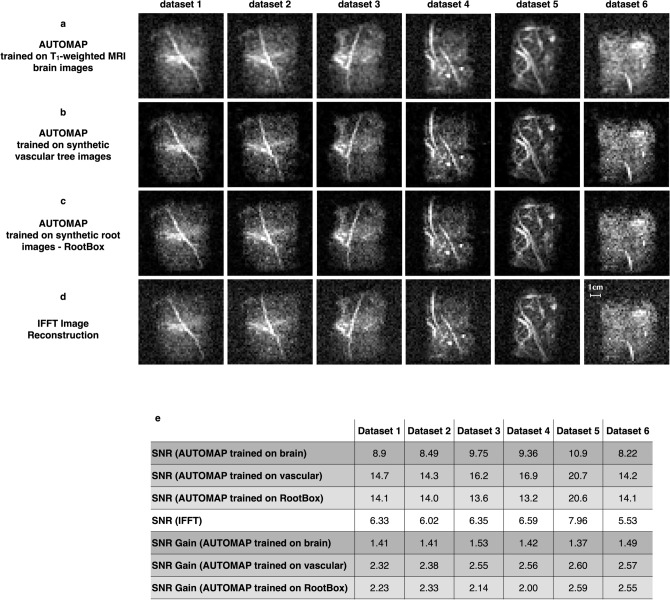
Reconstruction of sorghum dataset acquired at 47 mT using AUTOMAP trained either on T_1_-weighted MRI brain images, synthetic vascular tree Images, or synthetic root images. Six 2D projections of roots images (labelled dataset 1–6) extracted from six different roots’ samples are shown. All the 48 × 48 datasets were acquired at 47 mT. In the upper panel (a), the raw data were reconstructed with AUTOMAP trained on T_1_-weighted MRI brain images, the panel (b) are the same datasets reconstructed with AUTOMAP trained on synthetic Vascular Tree Images and finally the same datasets were reconstructed with AUTOMAP trained on synthetic root images in panel (c). The lower panel (d) shows the images reconstructed with the standard IFFT method. All the images were windowed to the same level for comparison. The scale of 1 cm represented one of the images is the same for all the 2D projections. (e) The table summarizes the mean SNR analysis of the 2D projections of the roots 48 × 48 datasets acquired at 47 mT.
